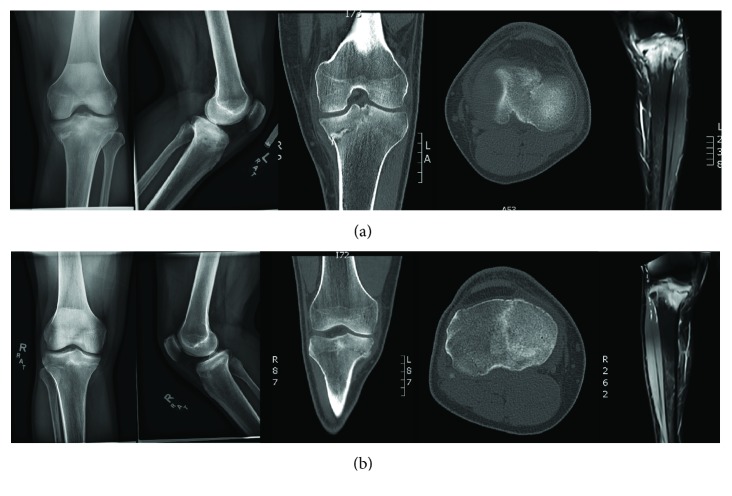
(a) Radiograhps, CT scan, and MRI of the left knee at the time of presentation demonstrate varus malalignment with medial sided proximal tibia stress fracture with extension to the articular surface and articular surface collapse. (b) Radiographs, CT scan, and MRI of the right knee demonstrate varus malalignment with medial sided tibia stress fracture without articular collapse.
